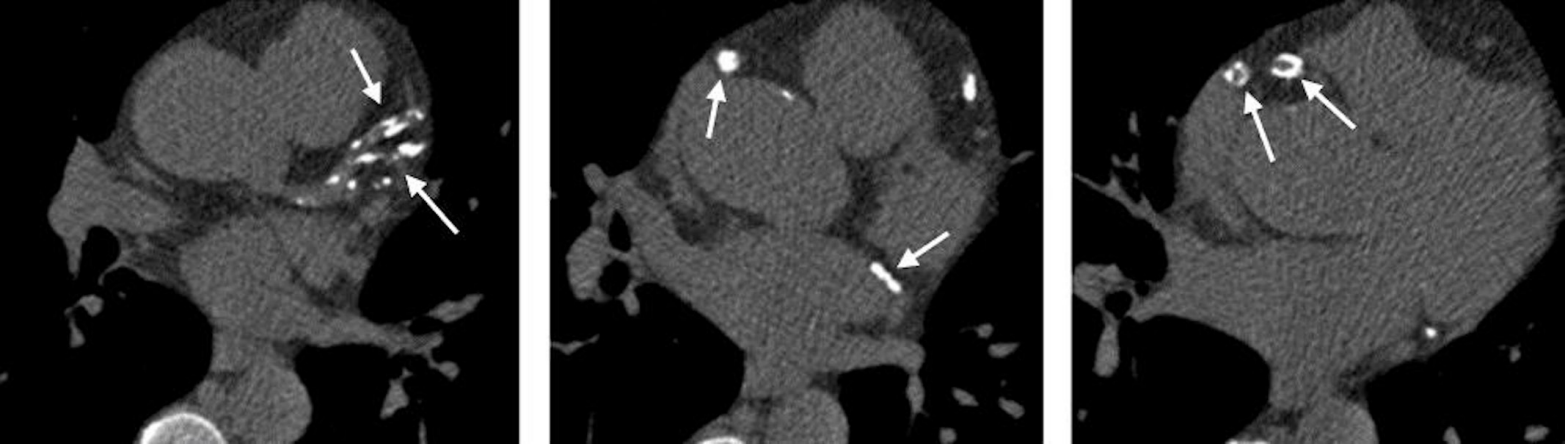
Cross-sectional, non-contrast CT images of the heart. Total coronary calcification score is calculated for each artery according to the density and area of calcification (arrows) observed in each artery. The sum of calcification scores in each artery gives a total coronary calcium score.
